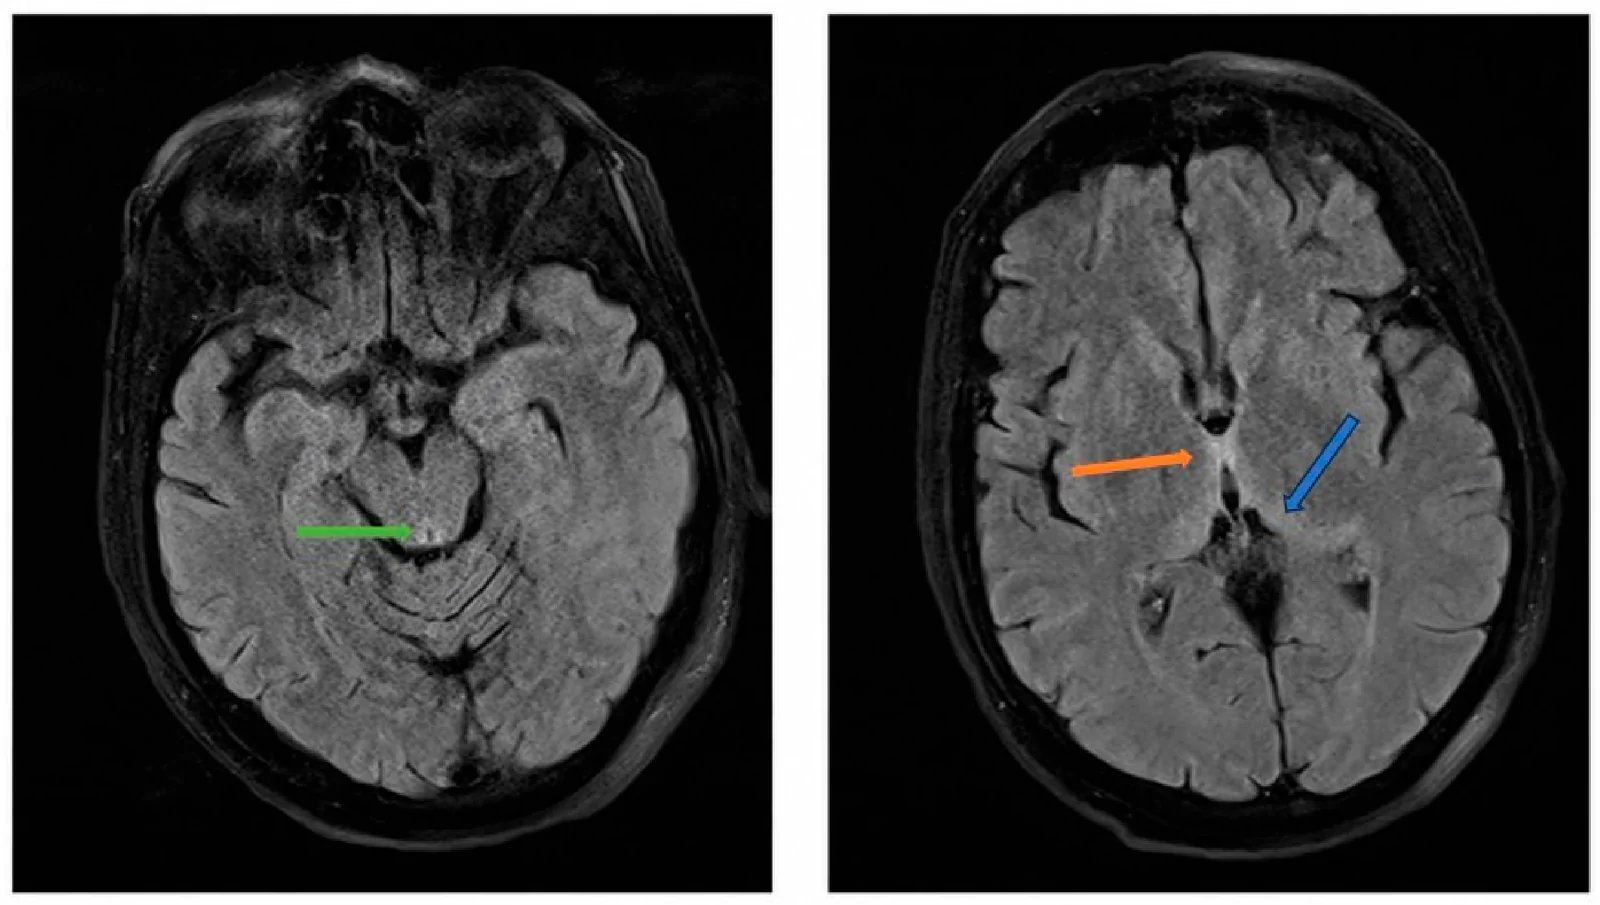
Early axial FLAIR MRI images obtained during hospitalization. Bilateral medial thalamic and periaqueductal FLAIR hyperintensities are shown. The green arrow indicates the periaqueductal FLAIR hyperintensity, whereas the orange and blue arrows indicate the bilateral medial thalamic FLAIR hyperintensities. In the subsequent clinical context, these findings were considered compatible with Wernicke encephalopathy.
